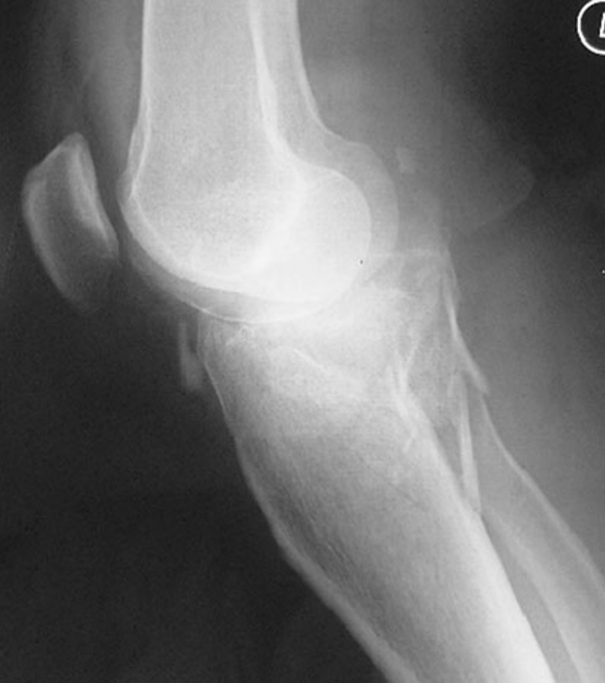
Schatzker type VI fracture, pre-op X-rays (LL)
